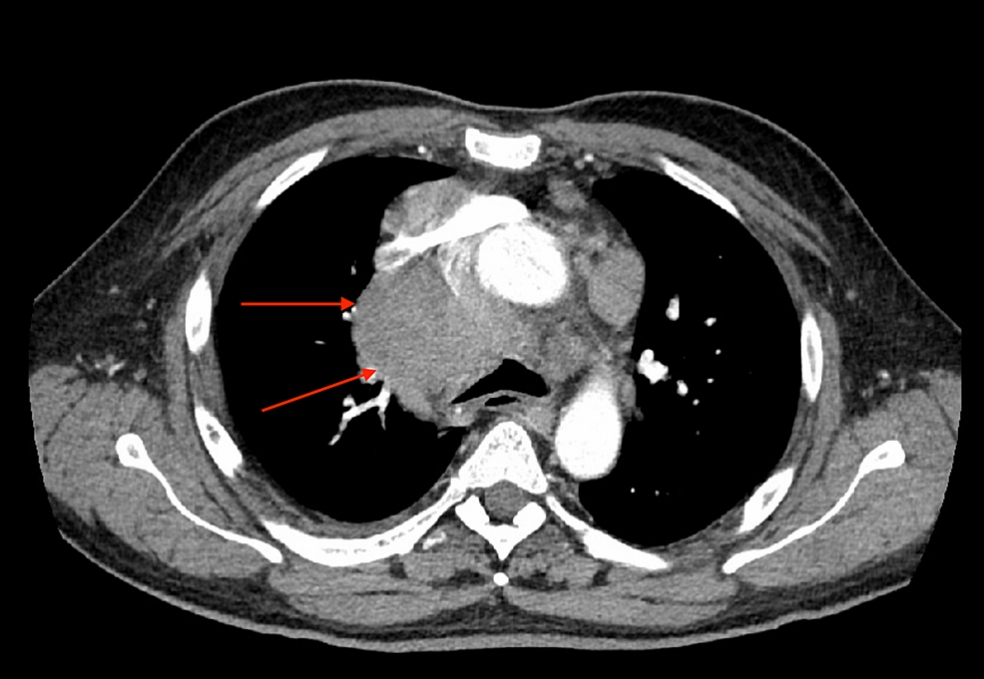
CT angiogram of the chest showing large mediastinal lymphadenopathy
